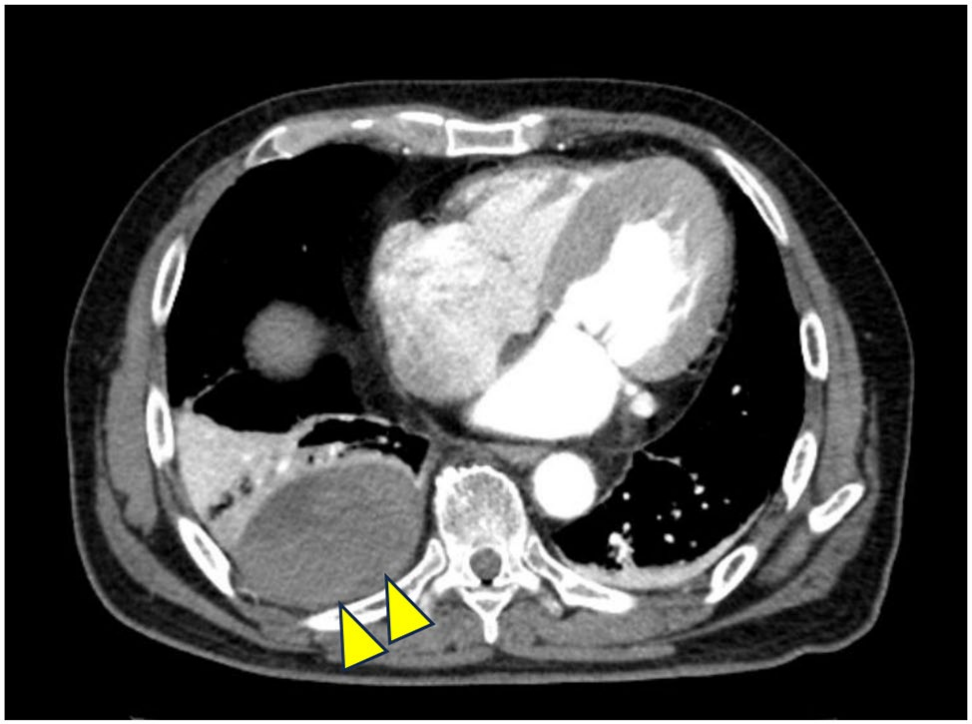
Chest CT findings: Hematoma 72 mm×52 mm×103 mm was observed immediately below the visceral pleura on the dorsal side of the right lower lobe. (yellow arrows)
